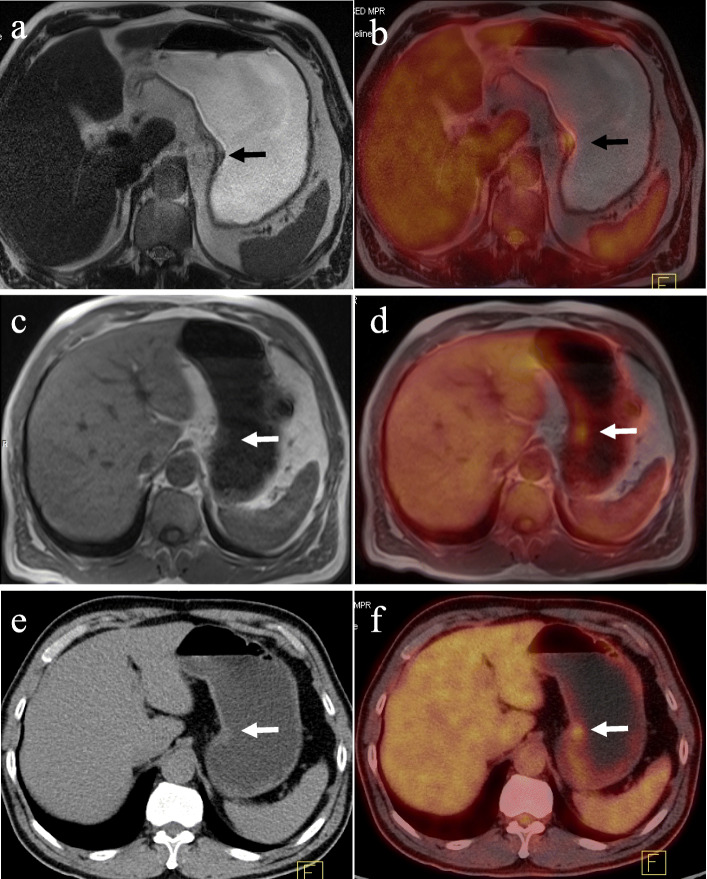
A 58-year-old man with gastric carcinoma at the lesser curvature of the stomach (arrow). Image artifacts were rated as“not hampering image evaluation” (score 2) and lesion conspicuity was rated as“excellently delimitable” (score4) for T2-HASTE image (a). T2-HASTE image showed an ulcer with crater shape and intact high-signal-stripe layer, which is a characteristic information of great value for staging, while this was not clearly visible on MRI-T1W and PET/CT images. Image artifacts were rated as “no artifacts” (score 3), lesion conspicuity was rated as“25–50% of borders definable” (score2) for both MRI-T1W (c) and CT images (e), image fusion quality was rated as“excellent” (score3) for PET/MR-T2W (b), T1W (d) and PET/CT (f) images
